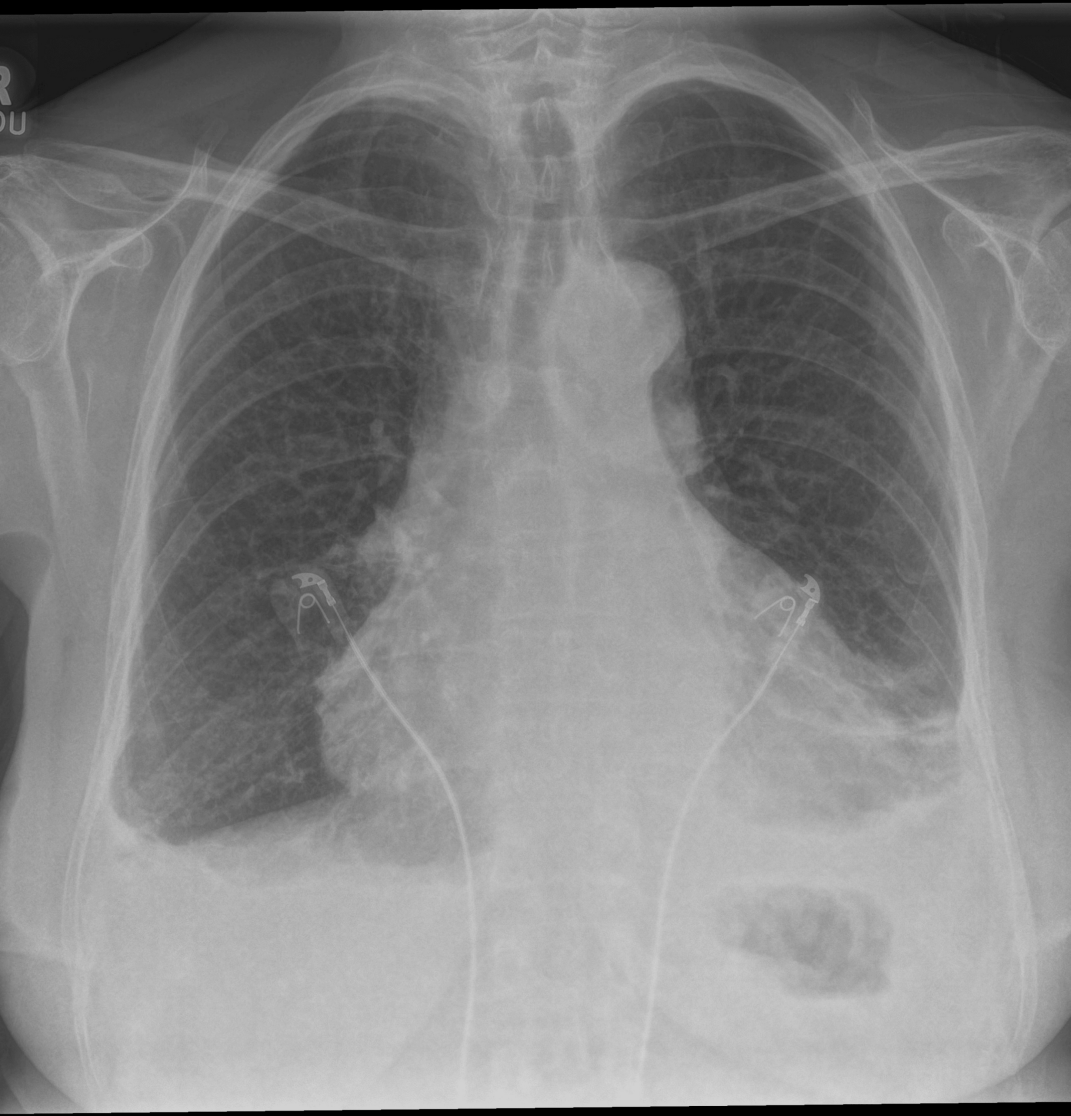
Chest X-ray showing bilateral pleural effusion
